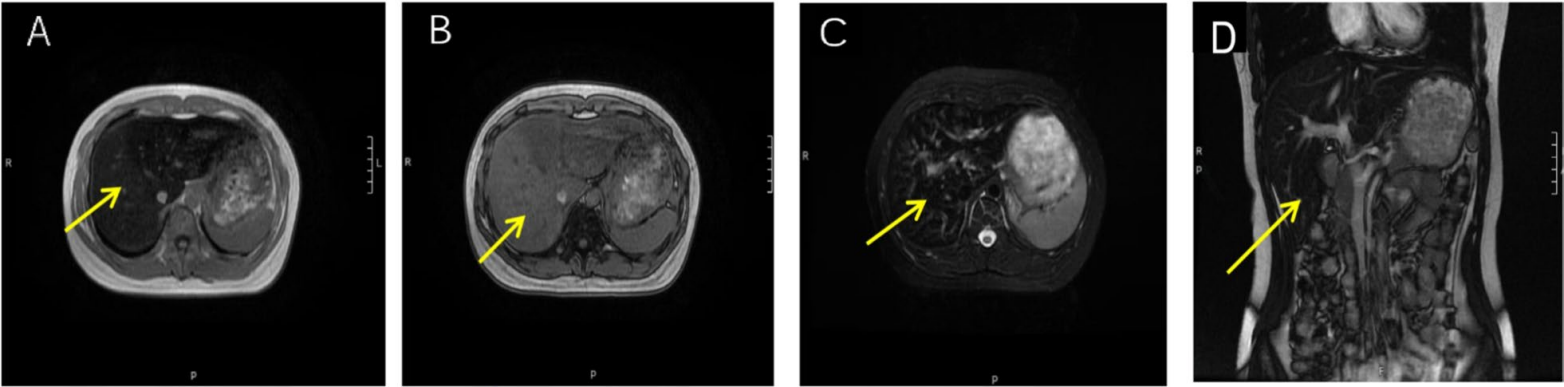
MR images of the upper abdomen in the axial (AX) and coronal (COR) views. A T1-weighted (T1W) in-phase images in the AX view showing that the liver signal is diffusely decreased. B T1W out-of-phase images in the AX view showing that the liver signal is diffusely increased. C T2-weighted (T2W) fat suppression (FS) images in the AX view showing that the liver signal is diffusely decreased. (D) T2W images without FS in the COR view showing that the liver signal is diffusely decreased. MR images indicate liver iron overload
